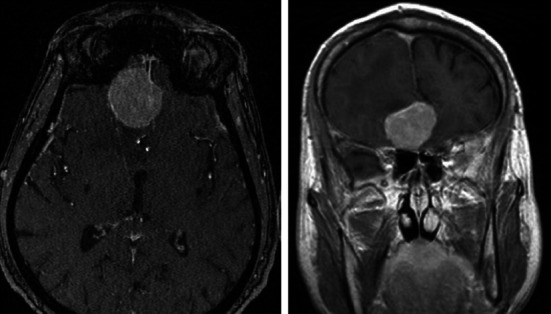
MRI (T2). Male patient aged 57—case number 2. History of anosmia and seizures for 2 years; personality distortion, worsening of verbal and logical contact on admission
